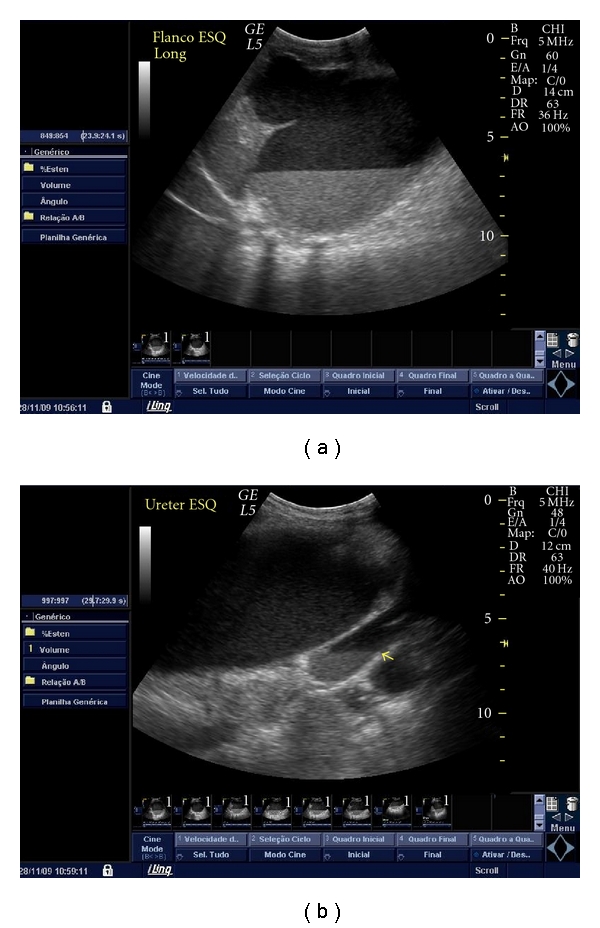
Abdomen ultrasound showing marked dilatation of the left pelvicalyceal system (a) and left ureter ((b) arrow), both containing hypoechoic fluid with posterior acoustic enhancement, and echogenic material deposited on the gravity-dependent portion with formation of fluid-fluid level.
